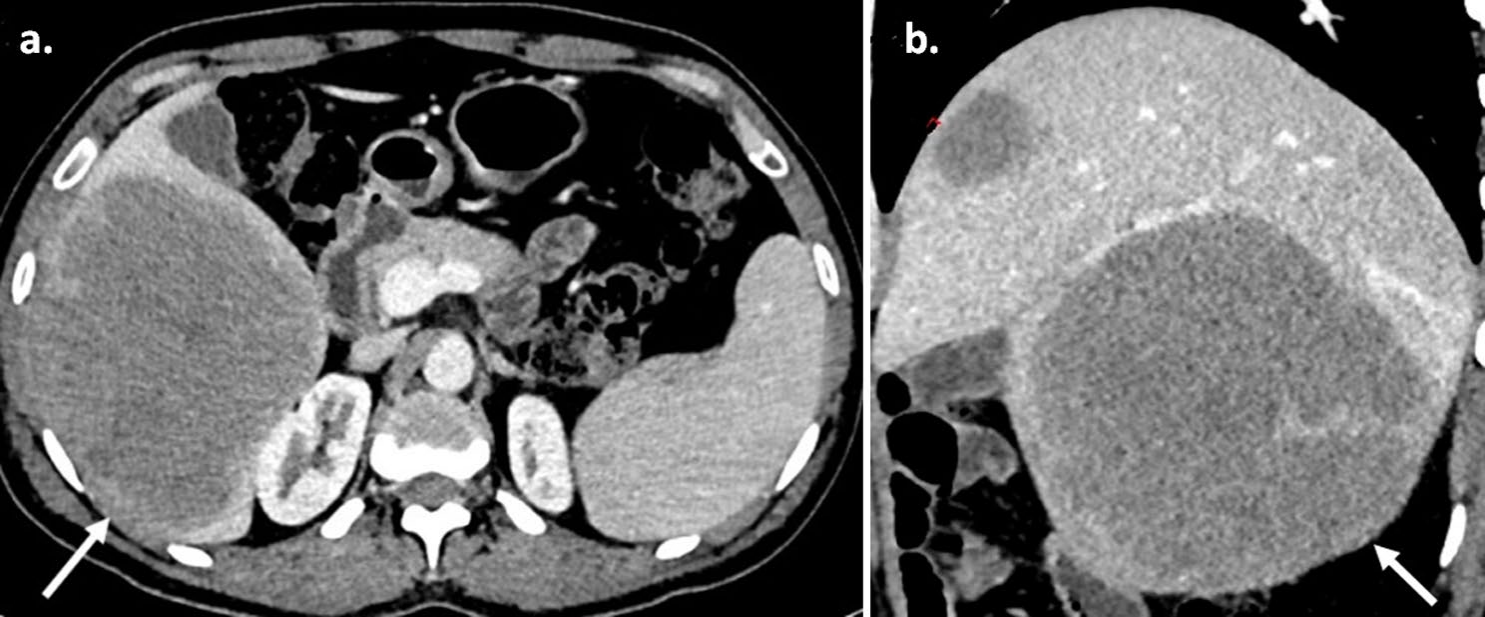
Contrast enhanced CT of liver. (a) Axial, (b) Sagittal CT images showing two hypoenhancing lesions (white arrows) with absence of perilesional edema
